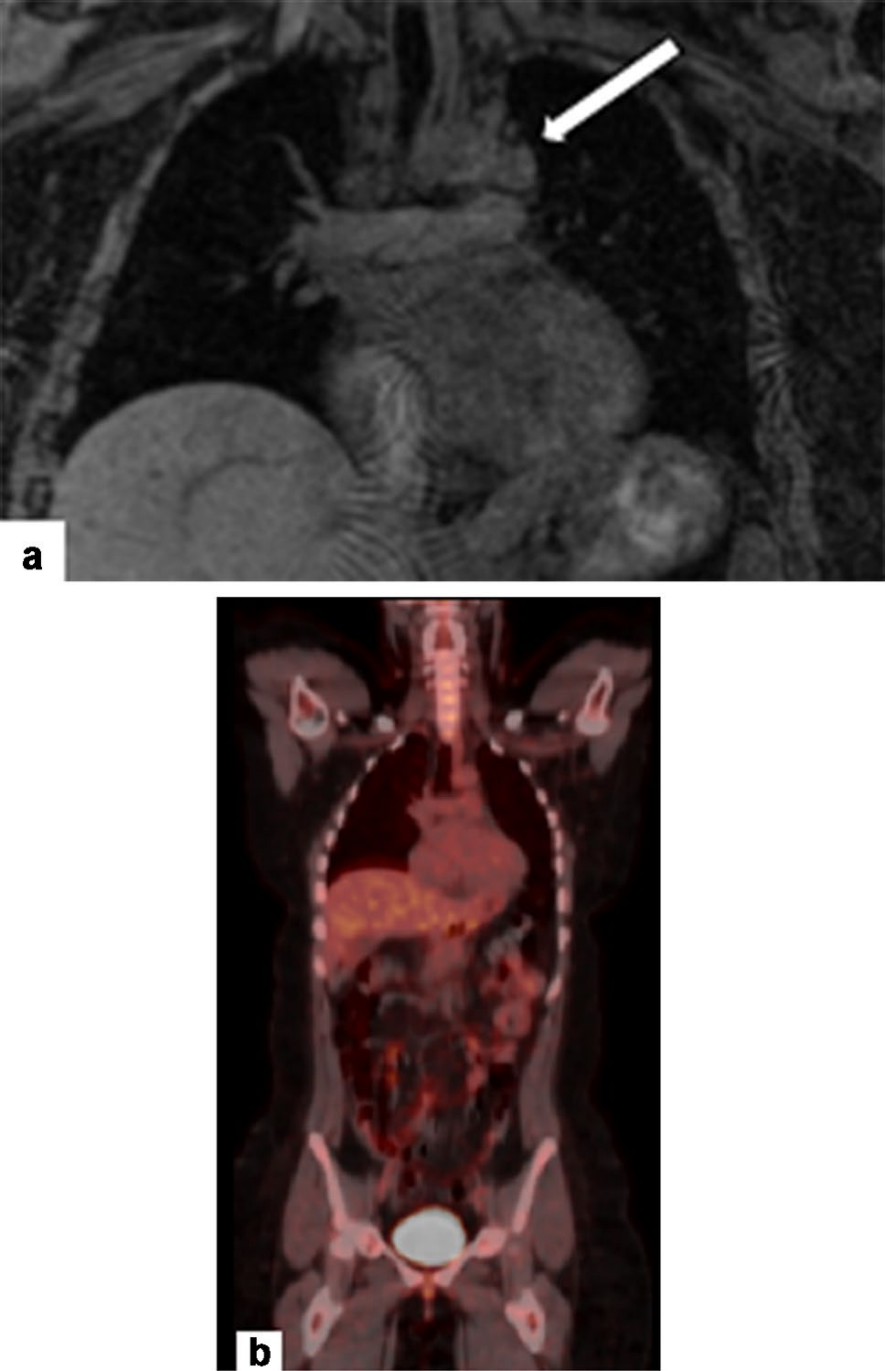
25-year-old pregnant patient in early first trimester, presenting with a palpable left supraclavicular mass. This was evaluated by ultrasound (not shown) which revealed an enlarged and abnormal lymph node corresponding to the patient’s palpable abnormality. Subsequent evaluation with fine needle aspiration and core biopsy, under ultrasound guidance, revealed Hodgkin’s lymphoma, at which time the patient was 8 weeks pregnant. Due to concern for fetal safety, staging was performed with a whole-body non-contrast MRI, instead of a PET-CT. a Coronal T1-weighted fat-saturated MRI demonstrated supraclavicular and mediastinal adenopathy (arrow). Chemotherapy was deferred until the second trimester. Maternal fetal medicine deemed no indication for early delivery based on her malignancy diagnosis. b Coronal fused images from the follow-up PET-CT after term delivery showed no evidence of disease, compatible with remission
